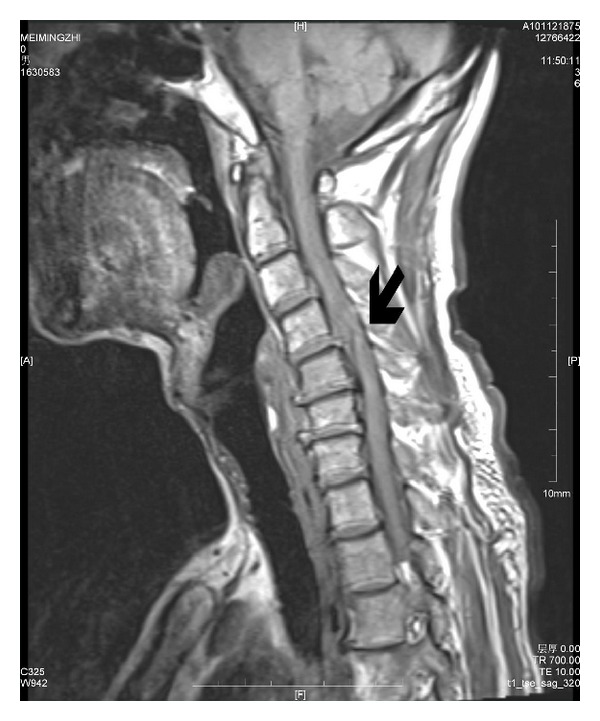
T2-weighted MRI demonstrates disc protrusion at C3-C4, C4-C5, and C5-C6, with spinal cord degeneration at 20 hours after gastrectomy. Arrow shows the lesion at the C4-5 level after the operation.
